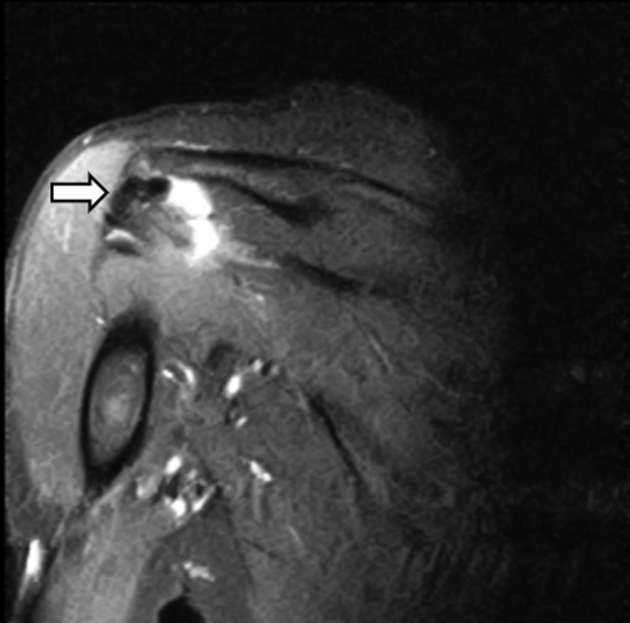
T2 inversion recovery coronal MRI of same shoulder from Figure 1. The image displays a postoperative MRI of a patient after superior capsular reconstruction with complete failure. The arrow denotes the dermal allograft still has some attachment to humerus and has balled up in posterior aspect of shoulder. There is no attachment to the glenoid on any coronal views thus demonstrating complete failure from the glenoid side. (MRI, magnetic resonance imaging.)
